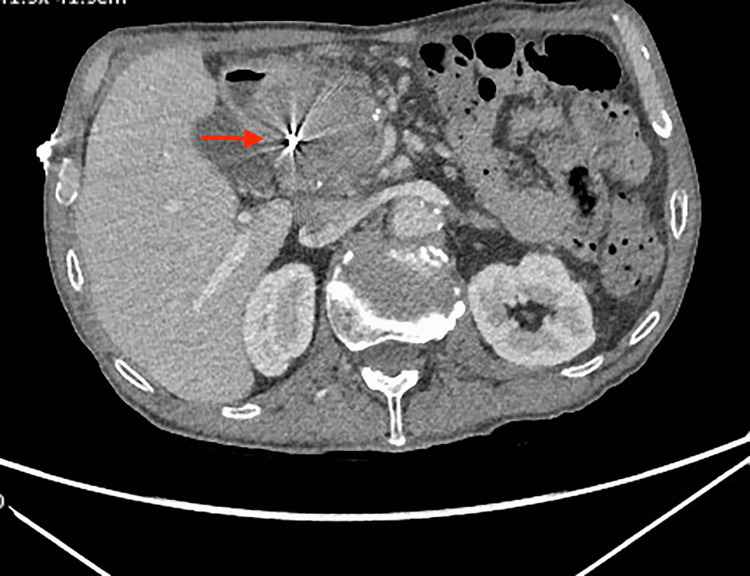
CT abdomen pelvis with contrast Axial CT image showing post-embolization coils (red arrow)
